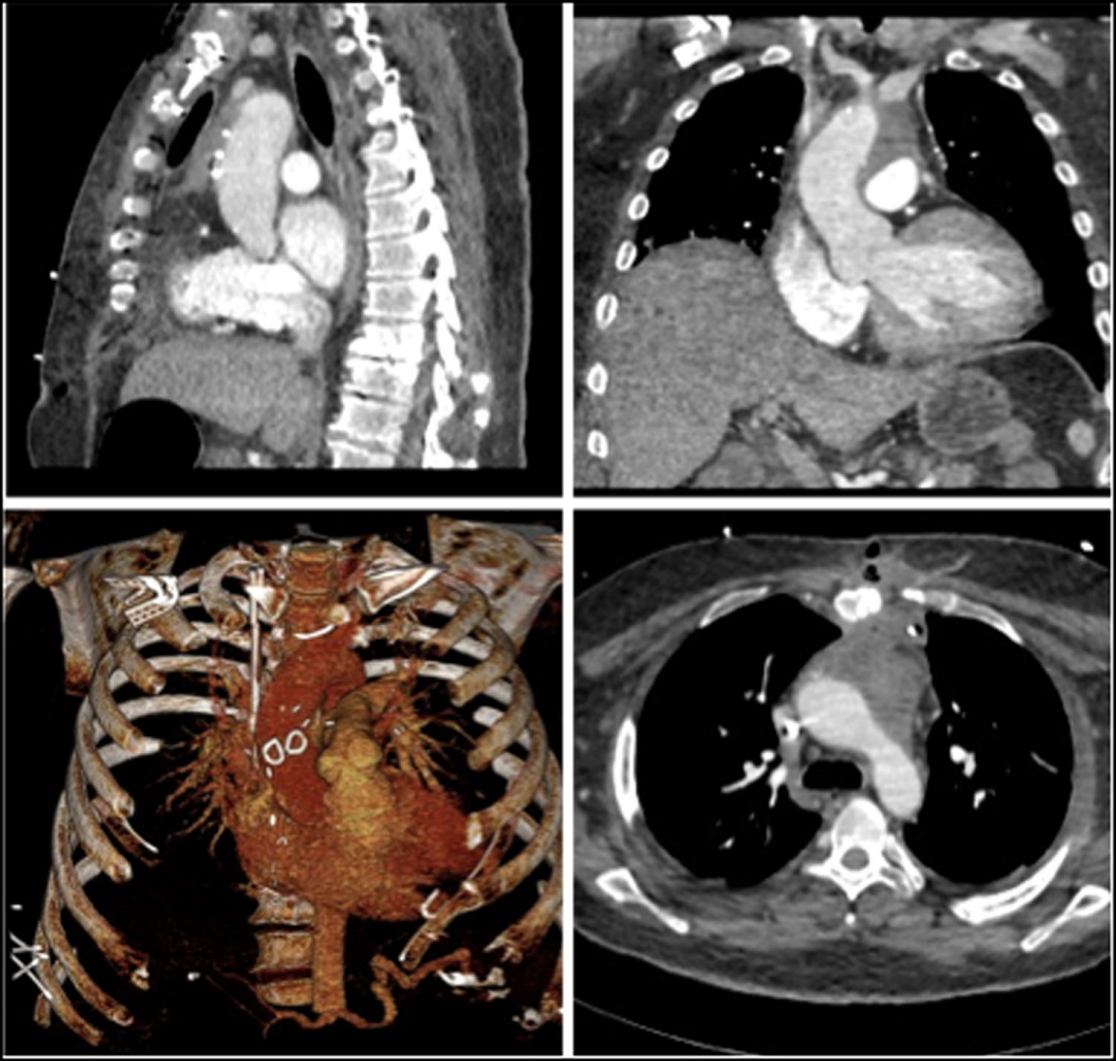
Computed Tomography images 2 days postoperatively demonstrating a normal aortic contour with resolution of the aortic pseudoaneurysm
